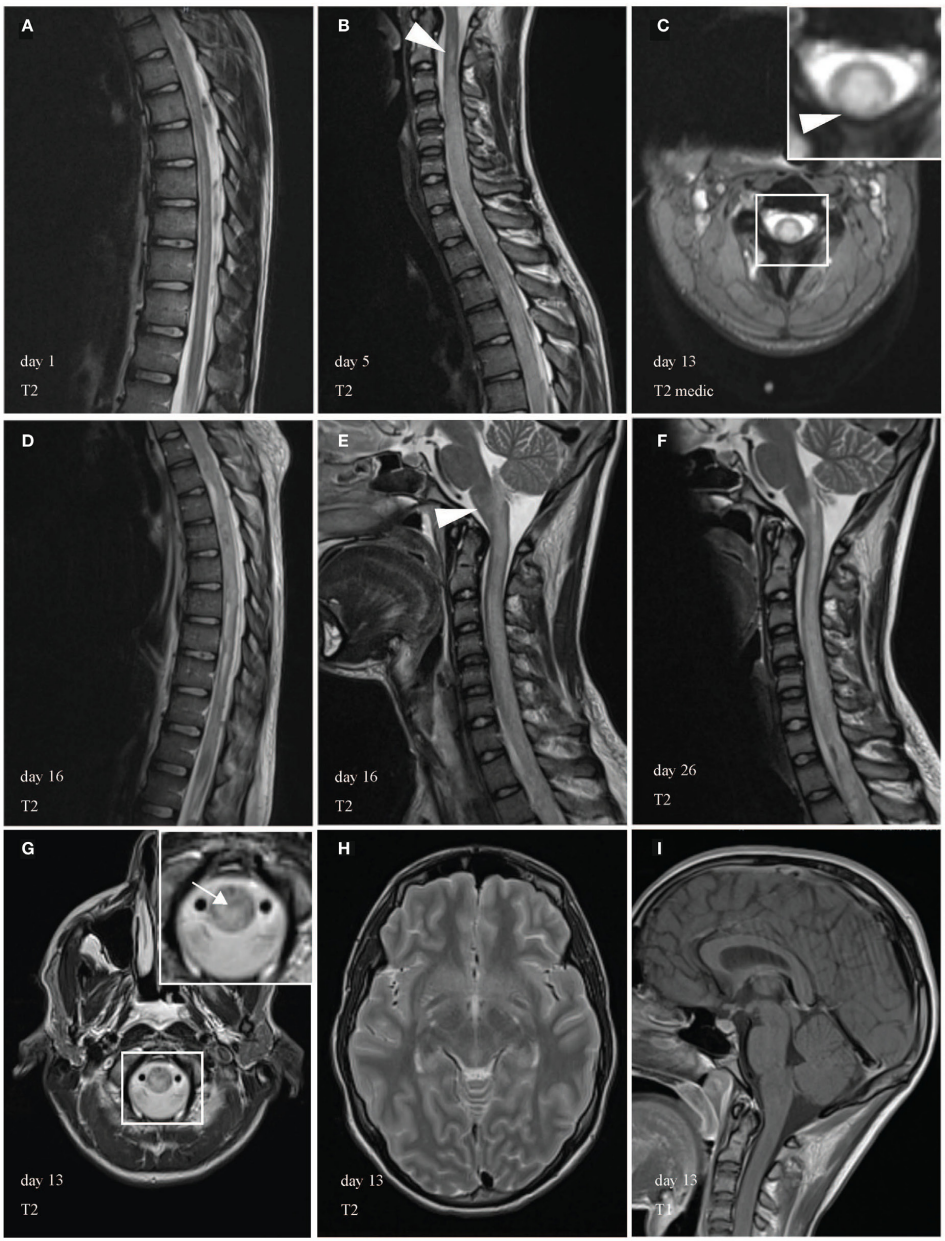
Magnetic resonance imaging (MRI) of the spinal cord during course of illness. MRI showed a longitudinally extensive transverse myelitis (LETM) of the cervical and thoracic spinal cord. In the beginning, the abnormal T_2_-weighted intramedullary signal hyperintensity appeared filigree, extended over more than seven vertebral segments and was most prominent in the anterior gray substance. (A) Few days later, MRI revealed cervicothoracic cord swelling a large-scale injury of the cord. During the course of illness, LETM expanded transversally (C) and craniocaudally (B,D) into the brain stem [(E,F), arrowhead]. (C,G) Demonstrate edematous changes in the cervical spinal cord and medulla in axial images (arrow). White box shows area of higher magnification. Brain MRI was unremarkable (H,I).
